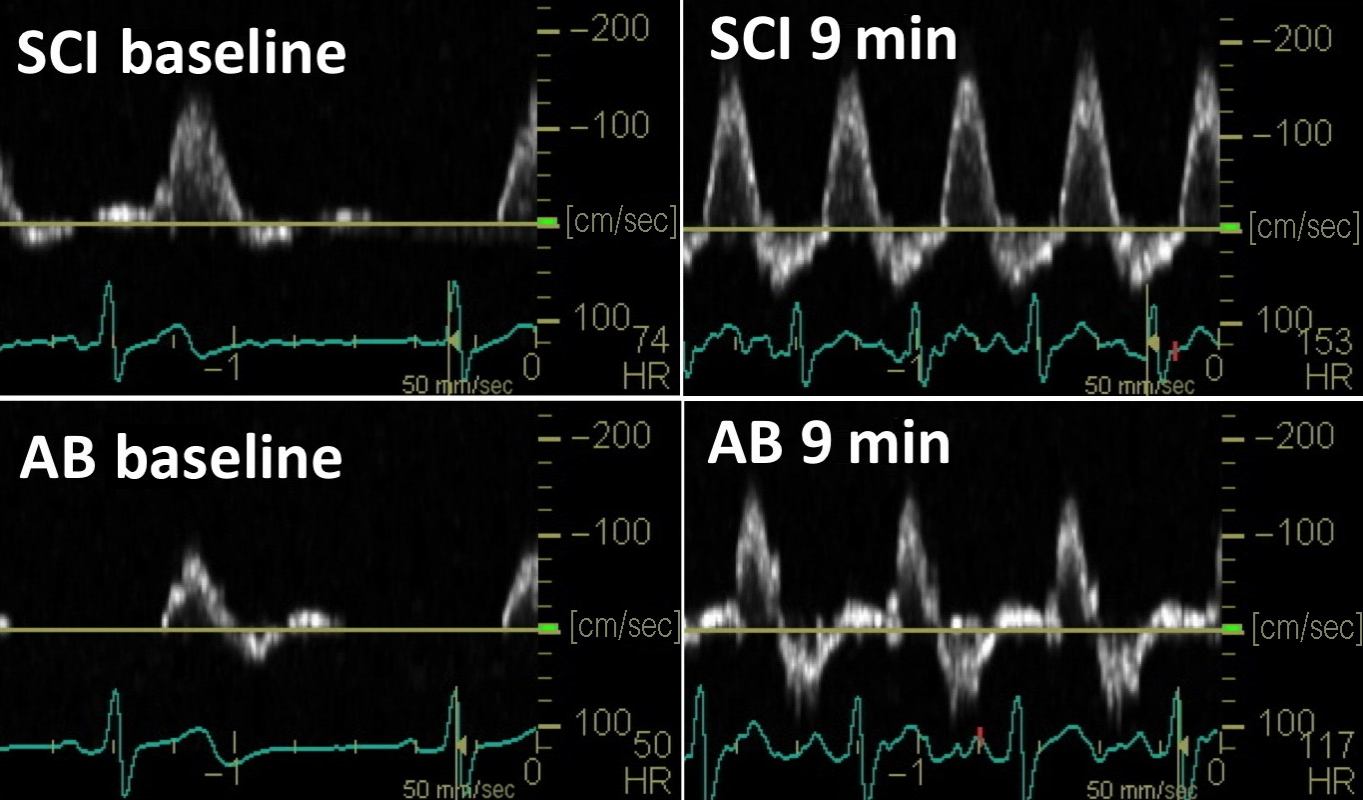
Arm-crank exercise intervention (Doppler Screen Capture). Superficial femoral artery blood velocity profiles at baseline and at 9-min of the arm-crank exercise intervention for an individual with spinal cord injury and an able-bodied control.
